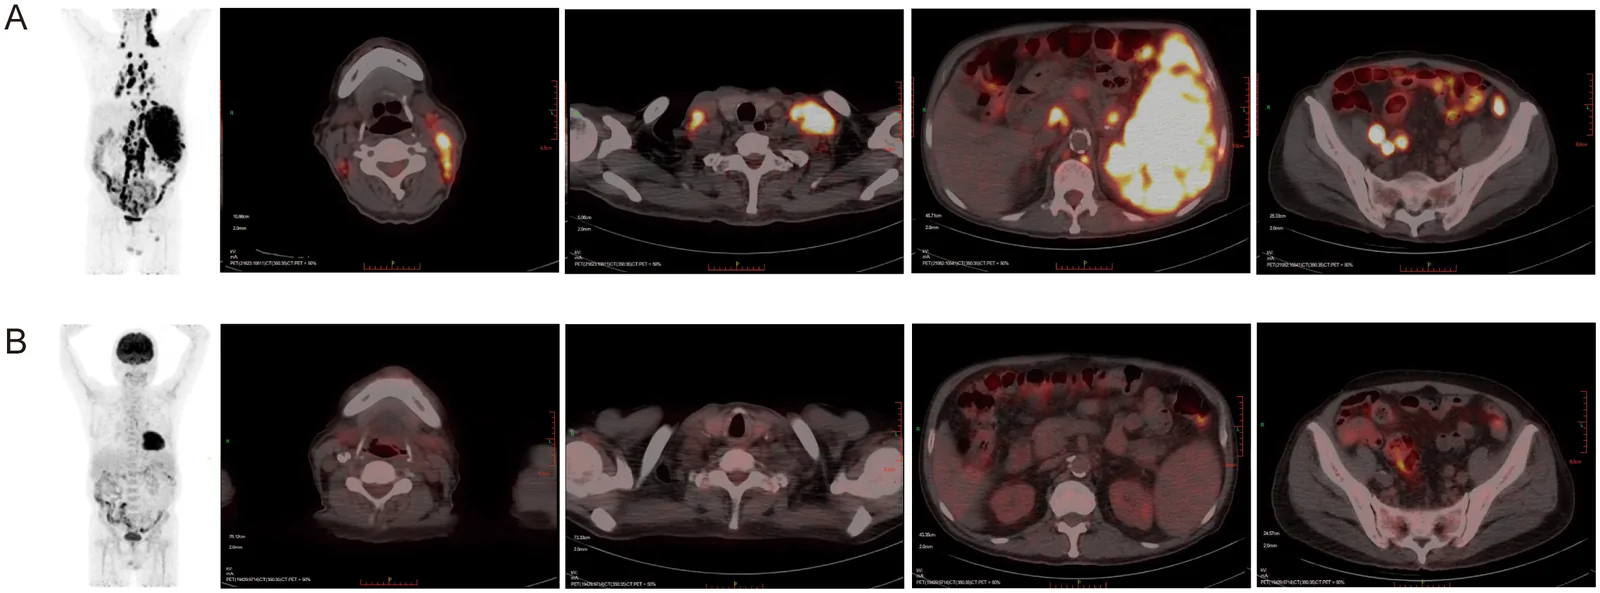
(A) The whole-body PET-CT February 6, 2025, revealed active disease progression. (B) A post-treatment whole-body PET-CT evaluation after 6 cycles treatment.
